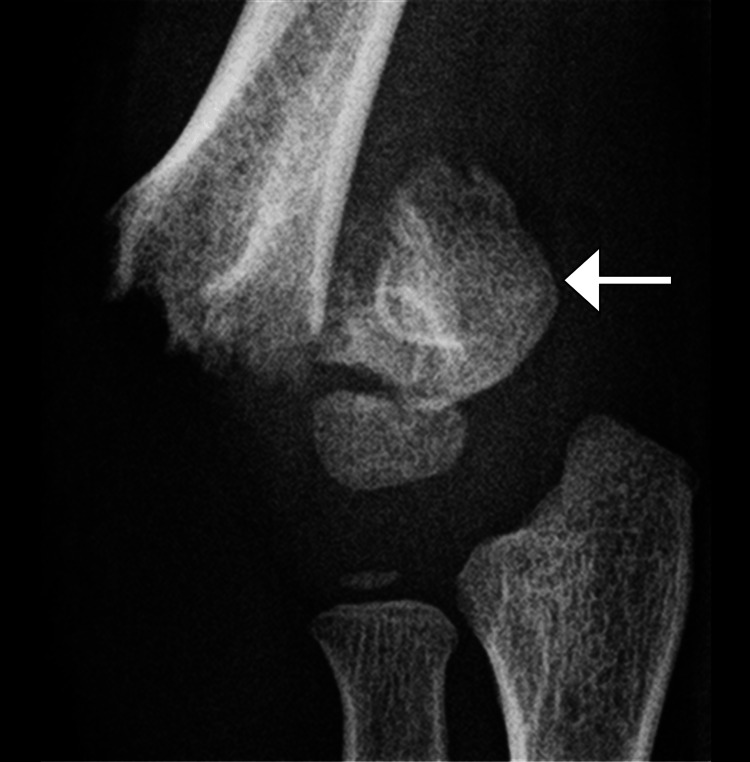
Lateral radiograph of the left elbow Lateral radiograph of the left elbow in a six-year-old patient exhibiting a Gartland Type III supracondylar humeral fracture. The image shows no cortical contact between the posteriorly displaced distal fragment and the proximal fragment (arrow).
